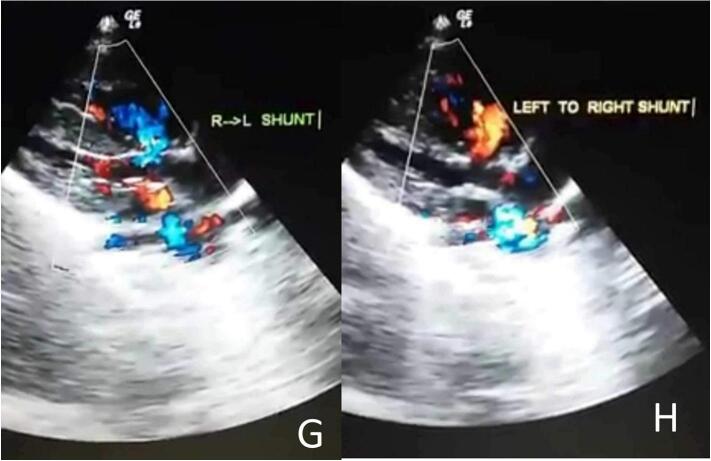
An echocardiogram Doppler image of a pulmonary valve showing severe pulmonary regurgitation and severe stenosis with a peak gradient of 95 mmHg (2-A). A parasternal short-axis view adjacent to the sternum, showing an Membranous ventricular septal defect (vsd), severe pulmonary arterial dilation, and a constricted ring in the area of the absent valve(2-B). The parasternal long-axis view, which is adjacent to the sternum, showed the type of defect along with malalignment of the outflow tract (2-C). The echocardiographic Doppler study demonstrates severe pulmonary regurgitation(2-D). The echocardiography shows a dilated pulmonary artery (2-E). The echocardiographic Doppler study demonstrates severe pulmonary stenosis with a maximum pressure gradient (PG max) of 95 mmHg (2-F). The parasternal long-axis in the color Doppler echocardiography reveals the presence of a right-to-left shunt (2-G). The color Doppler echocardiography's parasternal long-axis view reveals a significant defect causing a left-to-right shunt, characterized by a predominant flow from left to right (2-H).
